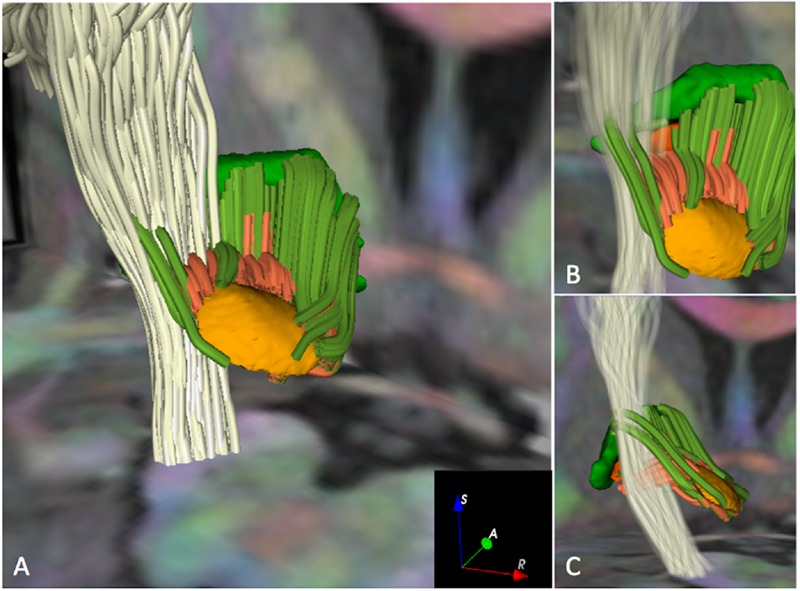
Crossings of the pyramidal pathway with subthalamo-pallidal and pallido-subthalamic fibers (MC-det approach) in HCP subject 100307. The figure shows 3D postero-superior views of the intersection of the pyramidal pathway (white streamlines) with fibers interconnecting the STN and the GPi (dark orange streamlines) and fibers interconnecting the STN and the GPe (dark green streamlines). An axial T1-weighted image at the level of the cerebral peduncles and a coronal T1-weighted image at the level of the anterior commissure with 3D surface models of the STN (light orange), GPi (dark orange), and GPe (green) are displayed for anatomical reference. A DEC map is overlaid on the T1-weighted images. The opacity and number of pyramidal fibers displayed in (A) have been reduced in (B) and (C) to enable the visualization of the crossings of the motor pathway with fibers interconnecting the STN with the GPi and GPe.
